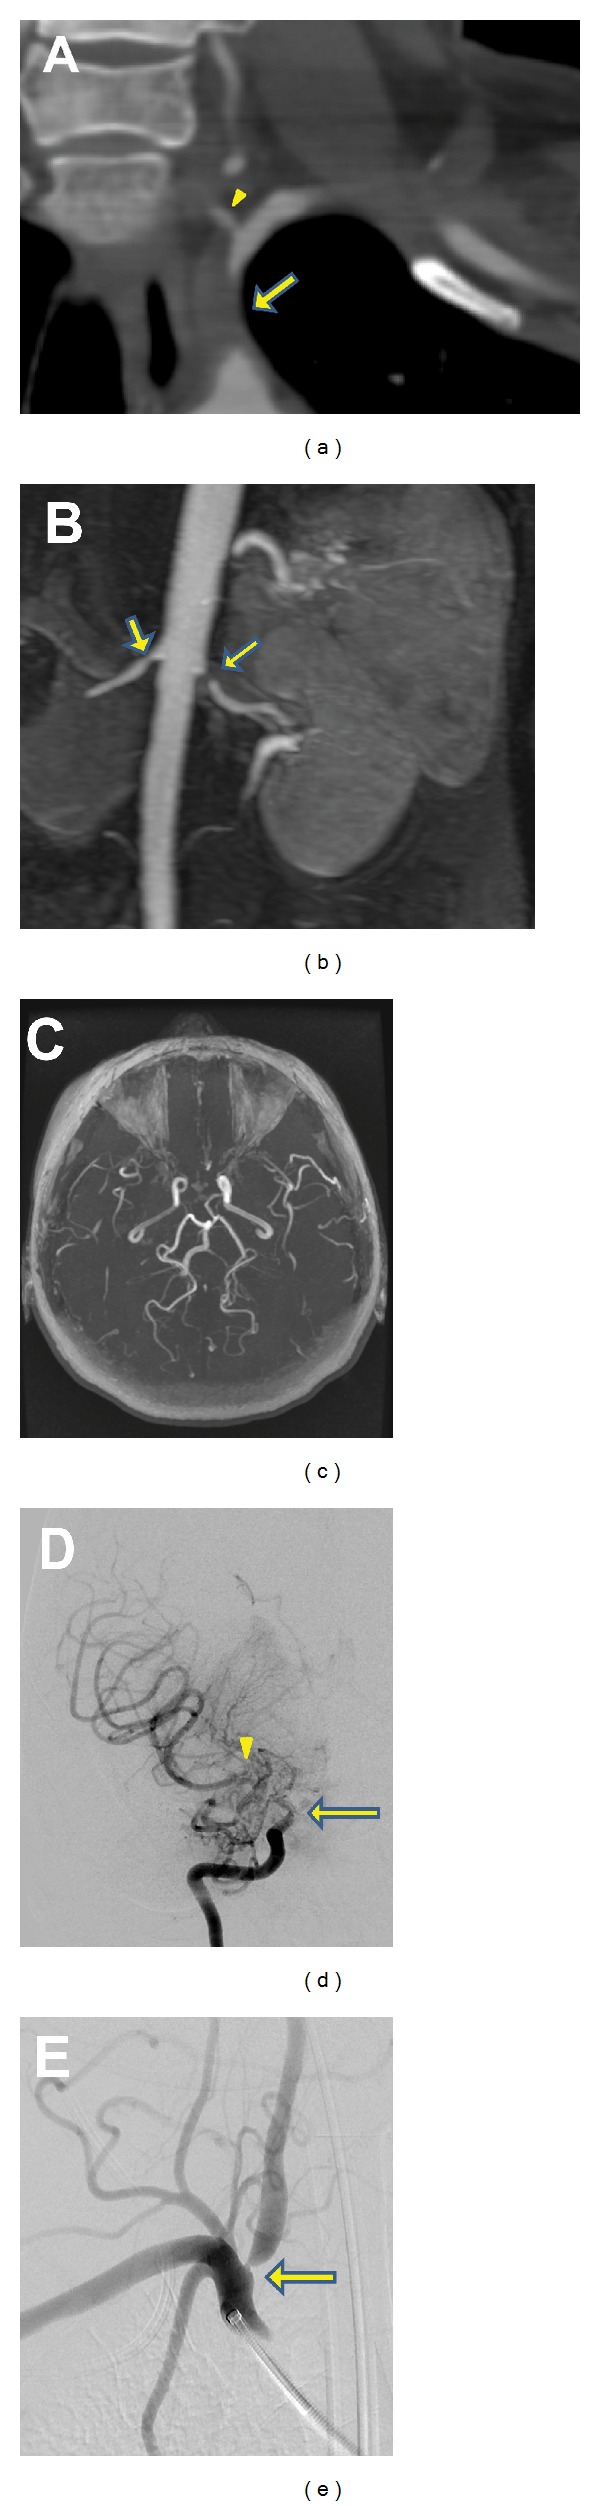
Multimodality imaging demonstrating stenoocclusive disease of intracranial and extracranial arteries. (a) CT angiogram chest demonstrating complete occlusion of left subclavian artery (arrow) with distal filling from left VA (arrowhead). Remainder of VA is out of plane of view, but patent distally. (b) MR Angiogram of abdomen demonstrating bilateral renal artery stenoses (arrows). (c) Time-of-flight MR angiogram of head showing absence of bilateral MCA vessels and decreased flow of both ICAs. The posterior circulation is relatively plethoric. (d) Conventional angiogram of the right ICA (AP projection) showing typical moyamoya appearance of intracranial occlusion of supraclinoid ICA (arrow) with reconstitution of the MCA via multiple small moyamoya collaterals (arrowhead). (e) Right subclavian digital subtraction angiogram demonstrating high-grade (>70%) right VA stenosis (arrow).
